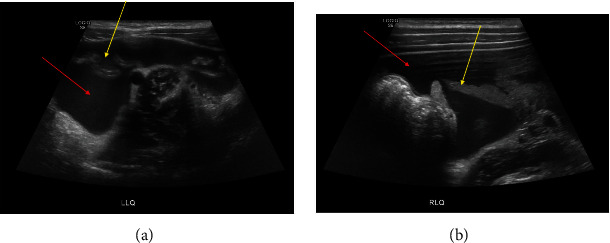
Ultrasound images of the left lower quadrant (a) and right lower quadrant (b) demonstrating ascites (red arrows) and omental thickening (yellow arrows).
